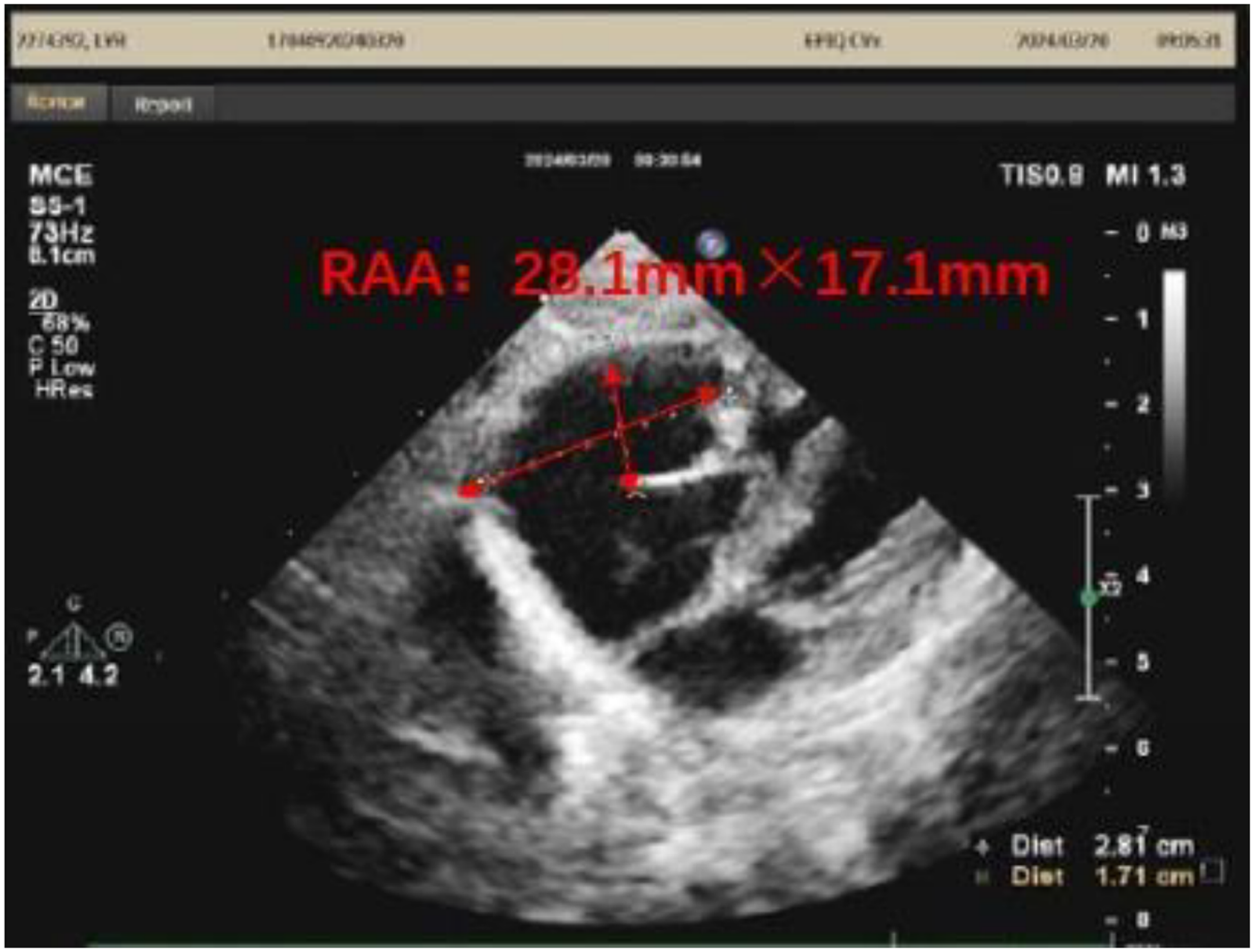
A transthoracic echocardiogram of the infant at 1 month of age. RAA, right atrial aneurysm.
